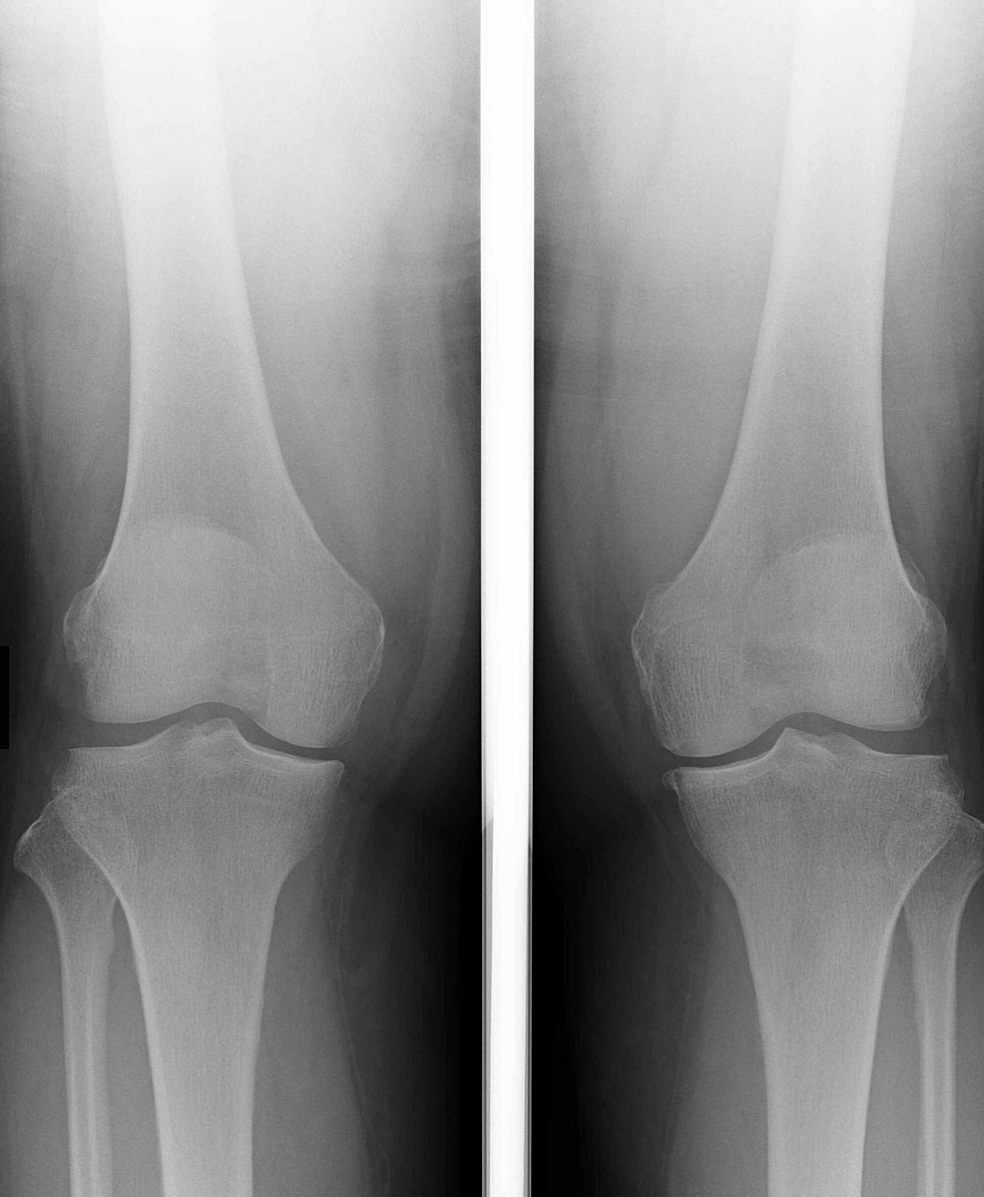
Preoperative X-ray The image showed a femorotibial angle (FTA) of 175 degrees and grade II Kellgren-Lawrence classification
